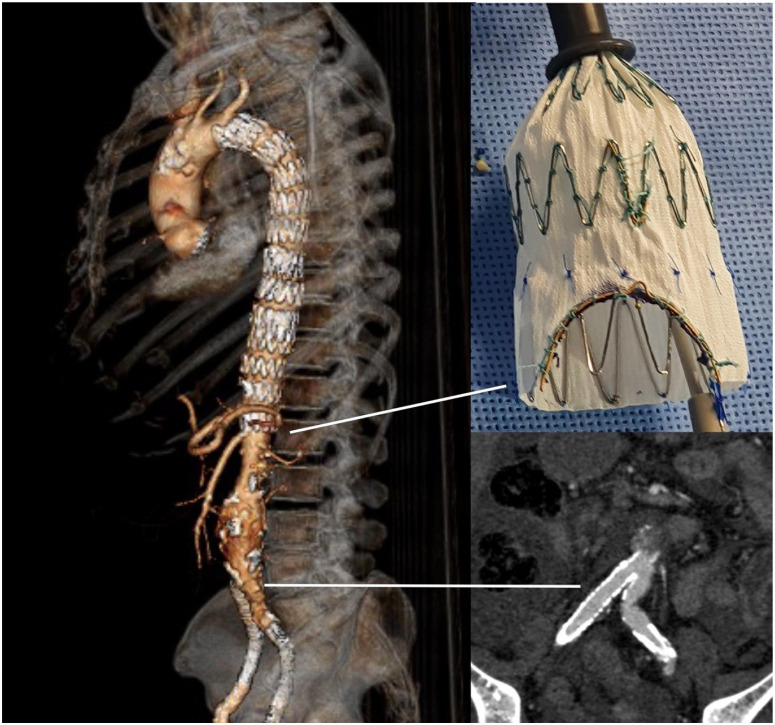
Three-dimensional rendering of the postoperative computed tomography scan with photograph of the scallop created in the distal end of the endograft for the celiac trunk and multiplanar reconstruction to show the patency of the covered stent implanted in the iliac artery with no sign of perfusion to the fenestration.
